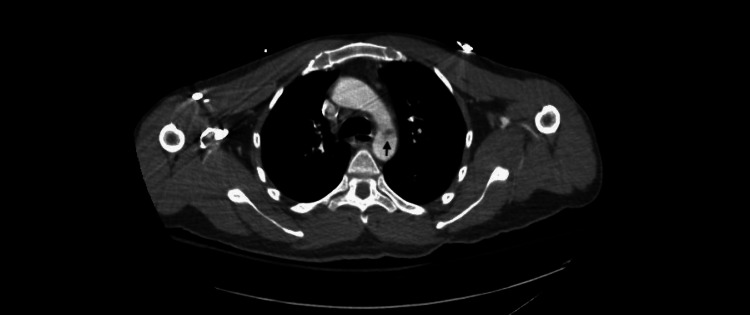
CT scan showing a filling defect in the distal aortic arch (arrow).
